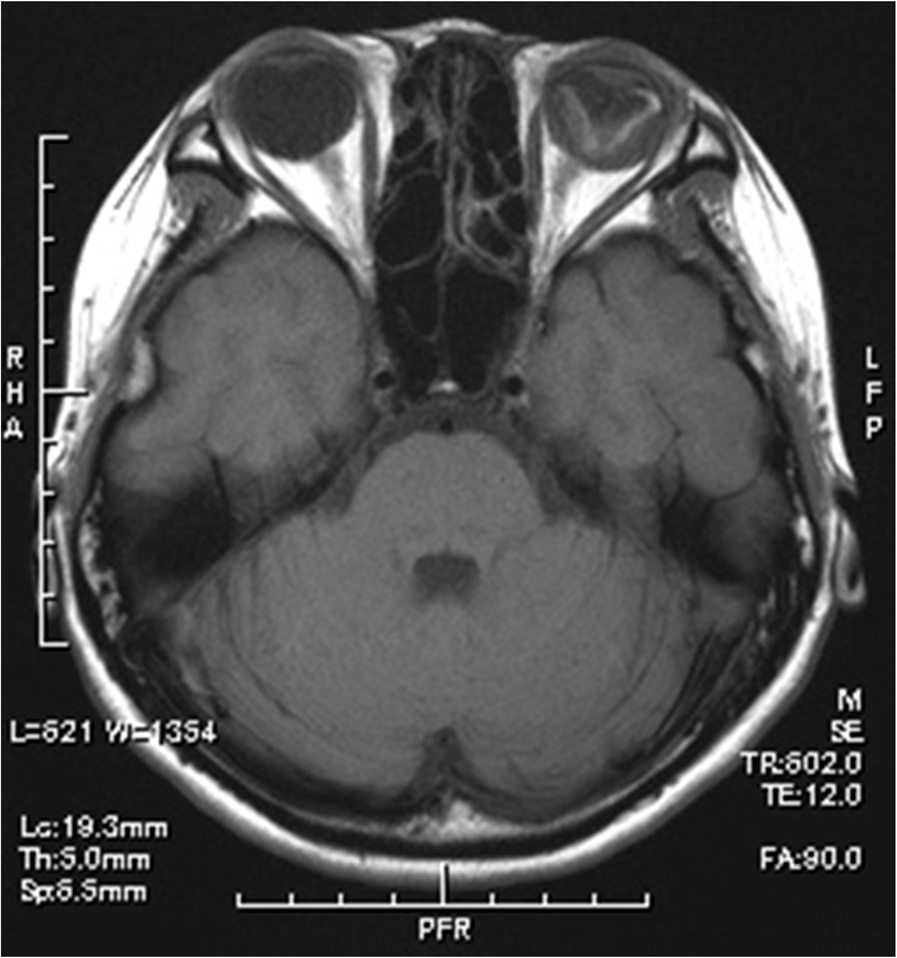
Axial view of follow-up MRI 10 years after radiotherapy (patient 1). Retinopathy of the left eye was observed.
